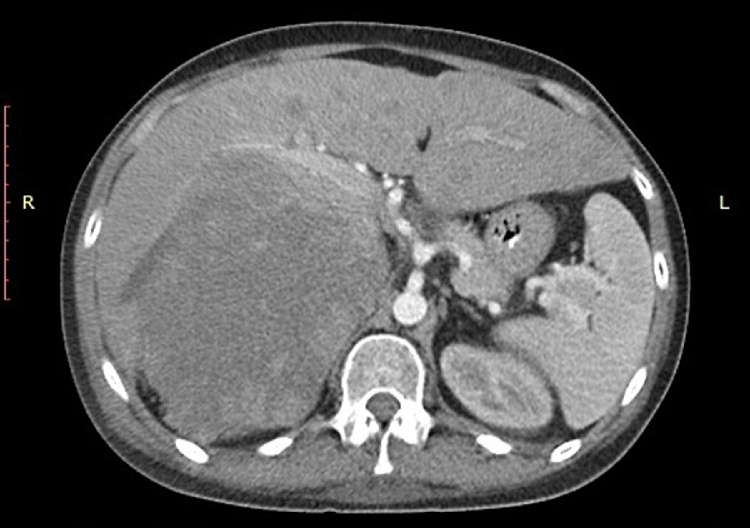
Abdominal CT scan demonstrating the presence of a large right adrenal cyst.
